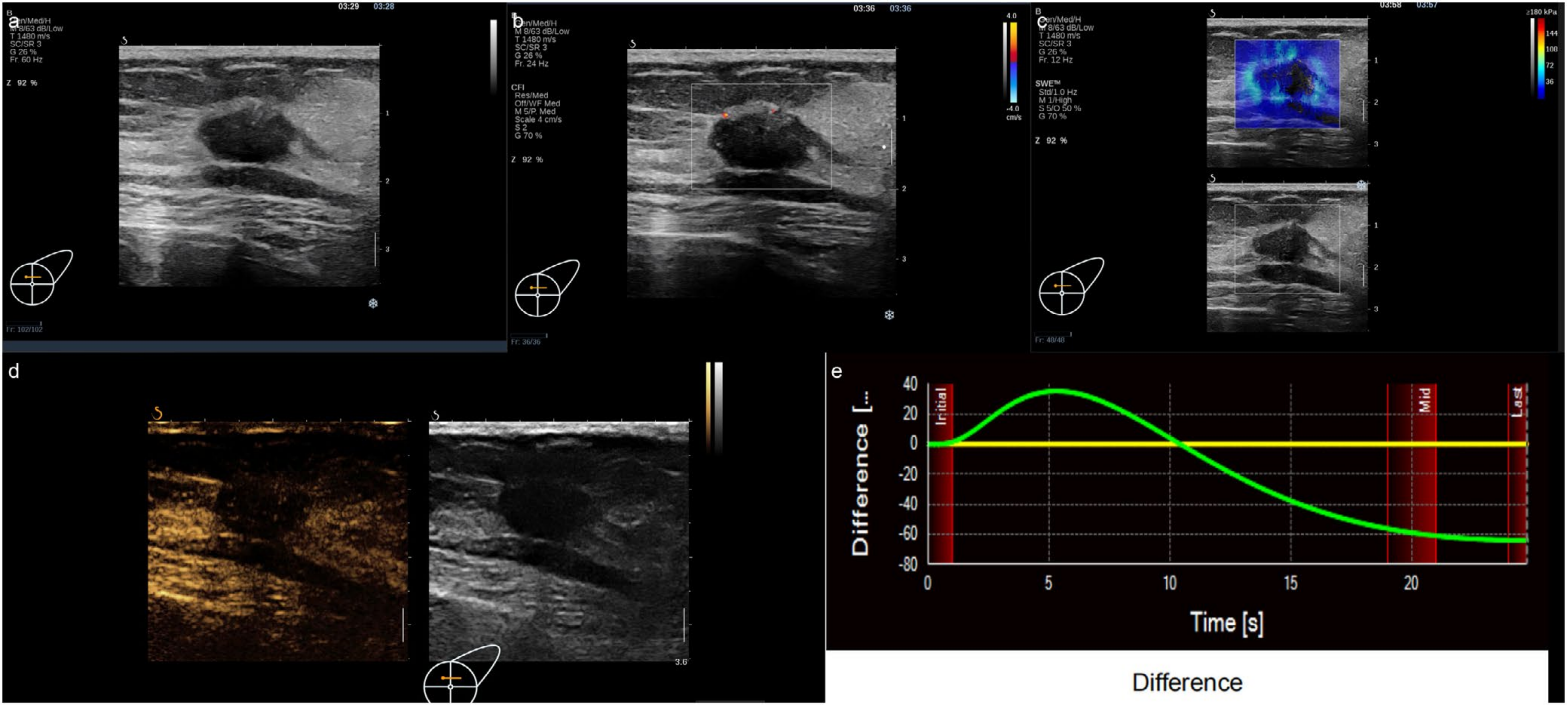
Multi‐modal ultrasound images of HER‐2 low breast cancer. The mass shows irregular shape, lobulated margins, calcifications, and Grade I flow. SWE reveals a black hole sign. CEUS demonstrates heterogeneous centripetal enhancement with a biphasic DVPC wave (initial rise followed by rapid washout).
